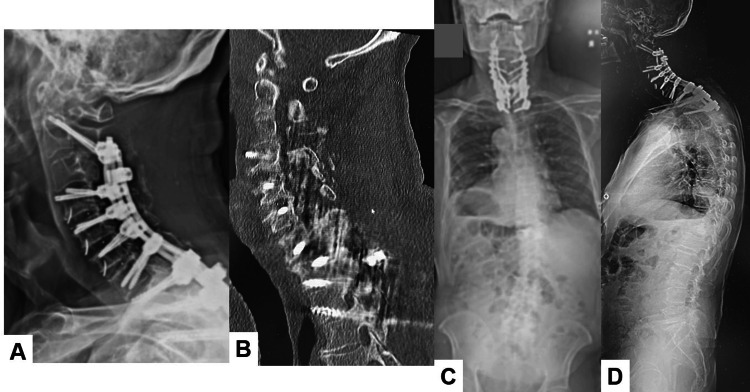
Final follow-up images. A. Cervical lateral radiogram. B. Mid sagittal cervical CT. C. Posteroanterior whole spine radiogram. D. Lateral whole spine radiogram. The spinopelvic parameters were as follows: OC2 angle 23, C2-7 kyphosis 26, T1 slop 45, cervical SVA 49.5 mm, PT 39, PI 49, lumbar lordosis 39, T1-4 angle 32, and T4-12 angle 33.
